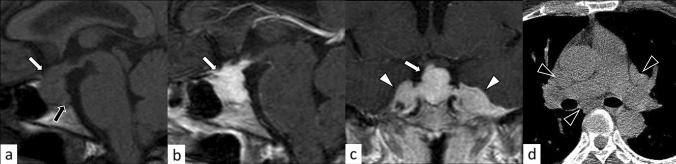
A 53-year-old woman with sarcoidosis. Enlarged pituitary gland and stalk with homogeneous and strong contrast enhancement are observed (a–c, white arrows). The posterior pituitary T1-weighted bright spot is absent (a, black arrow). The lesion is extending into the bilateral cavernous sinus (c, white arrowheads). Chest CT shows mediastinal lymphadenopathy, highly suggestive of sarcoidosis (d, black arrowheads)
